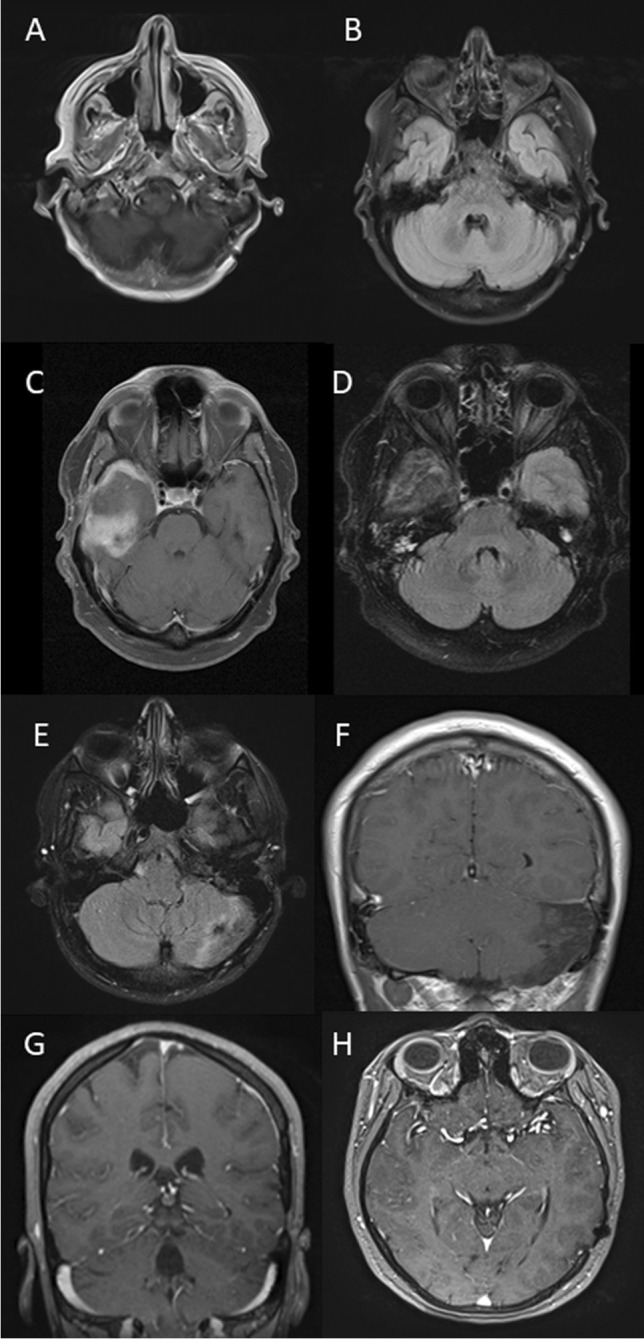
A, B Patient 1: axial gadolinium (Gd)-enhanced fat-saturated T1-weighted MRI shows a reduction of the mass lesions and of edema 4 years postoperatively. C, D Patient 2: axial FLAIR-weighted MRI shows significant reduction of cerebral edema in the middle and superior temporal gyrus. Axial Gd-enhanced T1-weighted MRI demonstrates also reduction of the solid mass in the inferior temporal gyrus 2 years postoperatively. E, F Patient 3: T2-weighted axial images show reduction of cerebral edema in the left cerebellar hemisphere. Gd-enhanced fat-saturated T1-weighted MRI further shows considerable reduction of contrast enhancement 4 years postoperatively. G, H Patient 4: T1-weighted gadolinium-enhanced axial and coronal MRI do not show a contrast-enhancing tumor any longer 5 year postoperatively
